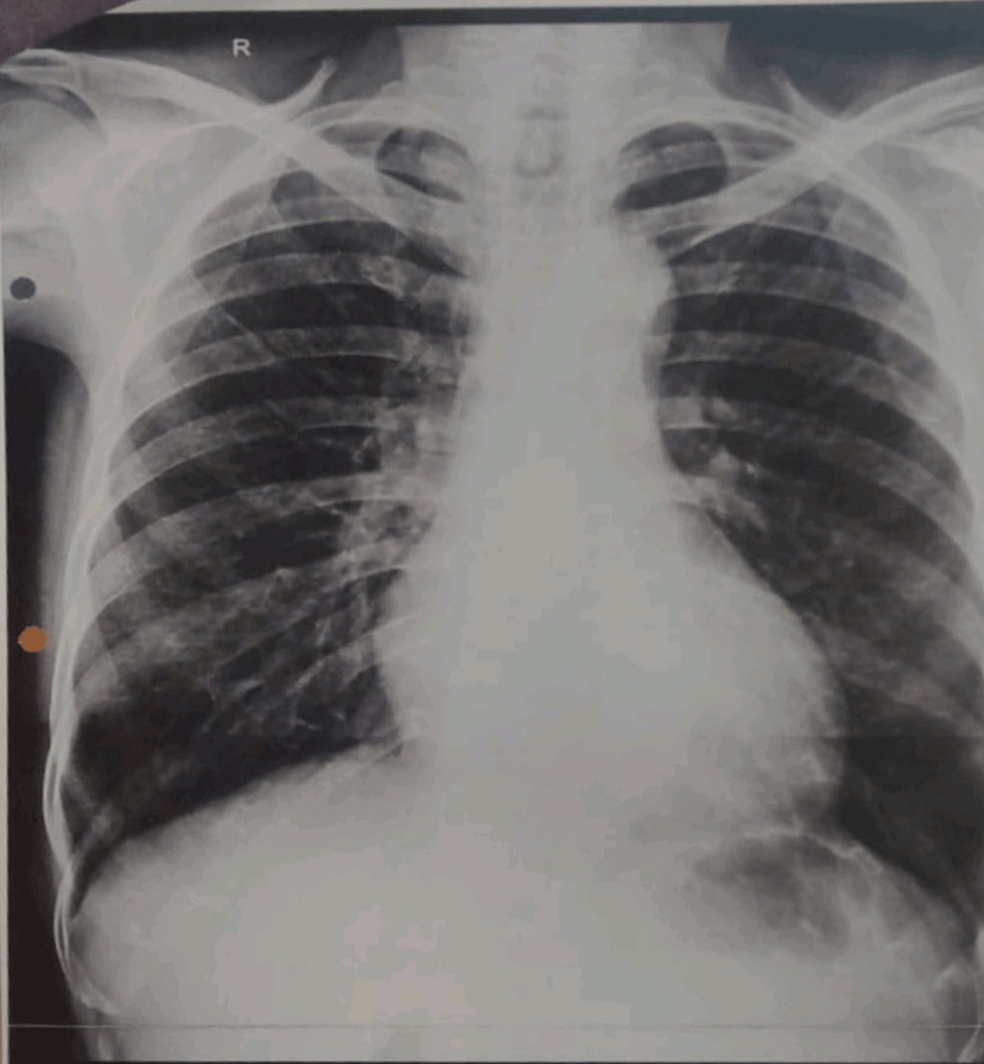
Chest X-ray Showing No Systemic Involvement
